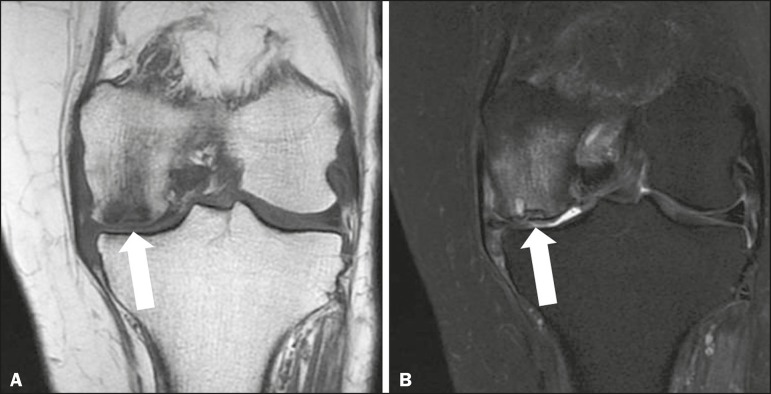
T1-weighted and fat-saturated T2-weighted sequences (A
